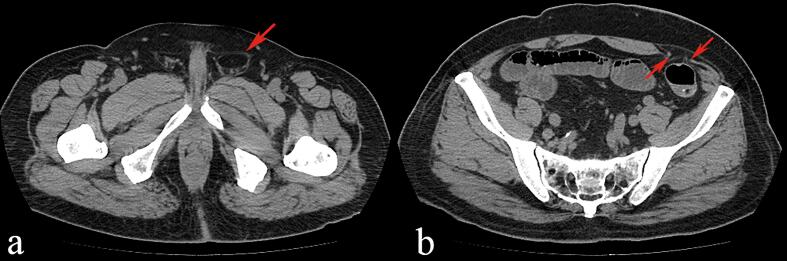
Computed tomography showing left inguinal hernia (1a) and left Spigelian hernia defect (1b) (arrowheads).
